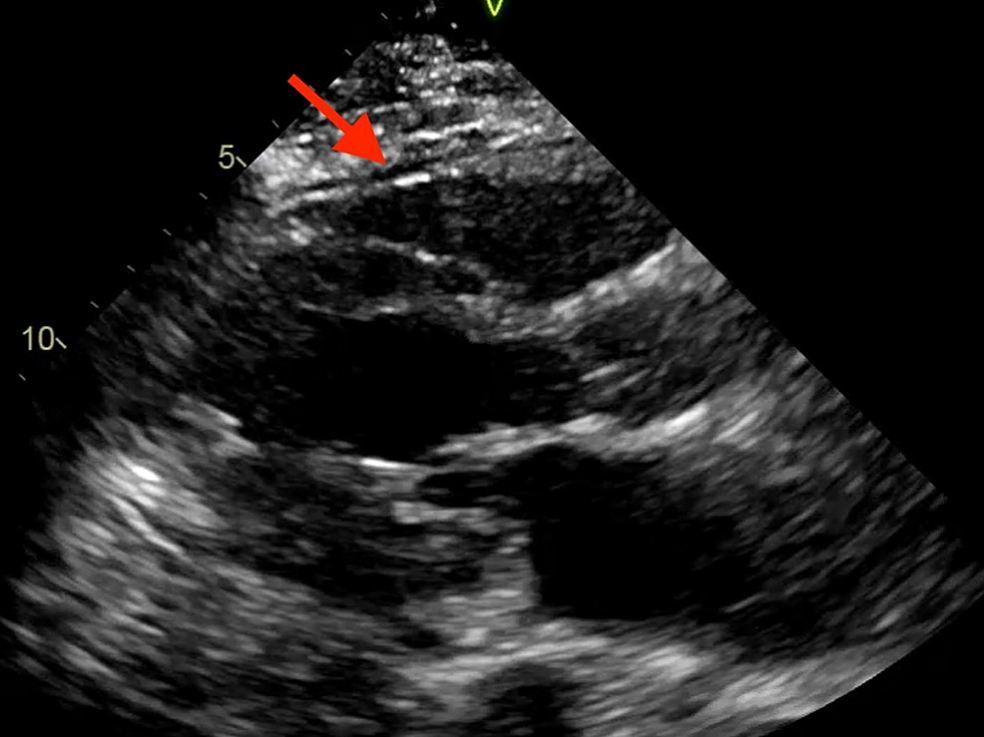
Transthoracic echocardiogram demonstrating small pericardial effusion Red arrow demonstrating the small pericardial effusion
